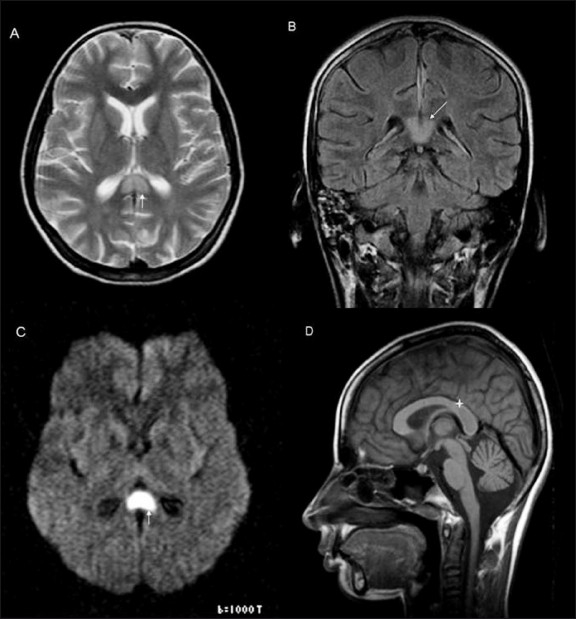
Axial T2W (A), coronal FLAIR (B), and diffusionweighted (C) images show a hyperintense, well-defined lesion (white arrow) in the splenium of the corpus callosum with a thin rim of surrounding non-hyperintense white matter. The same lesion on the sagittal T1W image (D) shows hypo to isointense signal (star)
